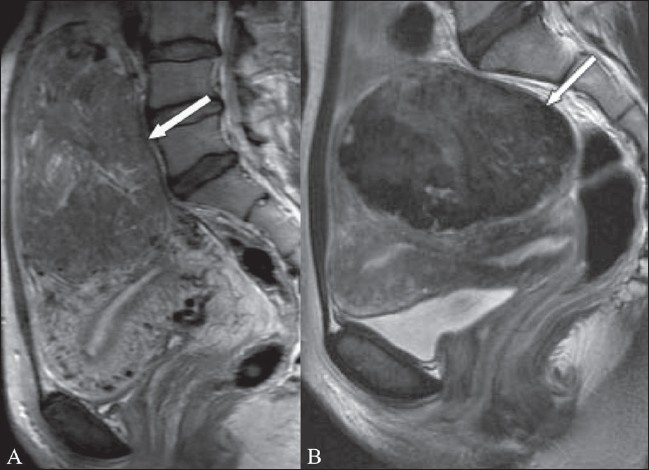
A 37-year-old woman with menorrhagia. Sagittal T2W MRI (A) shows an 18-cm, broad-based, subserosal fibroid (arrow). Repeat sagittal T2W image (B), 3 years post-UAE shows good response to treatment: the fibroid has reduced in size, now measuring 10.5 cm in AP dimension (arrow)
